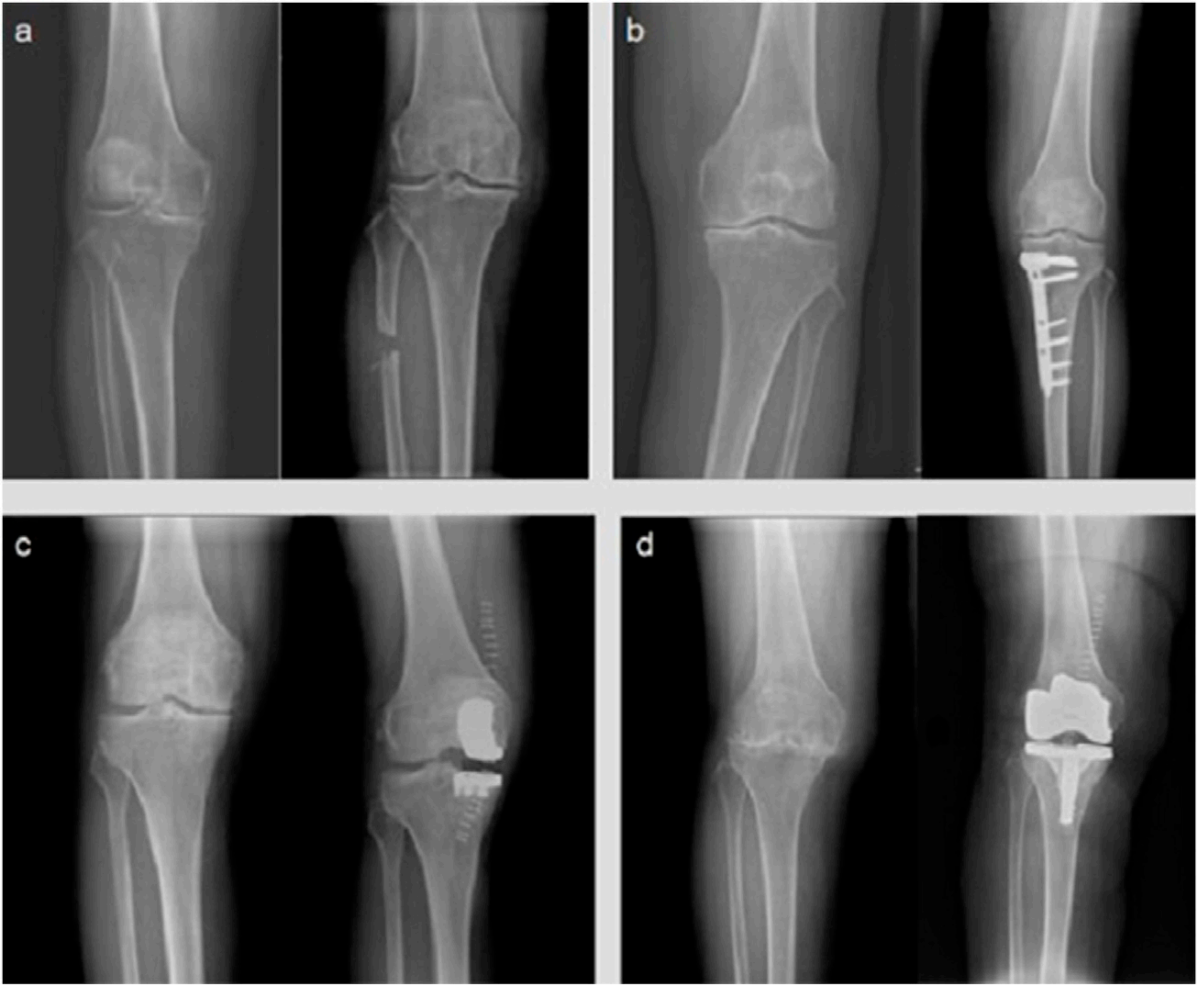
Digital radiographs of knee OA patients. Here is the comparison of the main surgical methods for the treatment of knee osteoarthritis before and after different surgeries (left: before surgery; right: after surgery) operation, each picture shows the digital radiography before and after the operation: (A) proximal fibula osteotomy; (B) high tibial osteotomy; (C) unicompartmental knee arthroplasty; (D) total knee arthroplasty.
